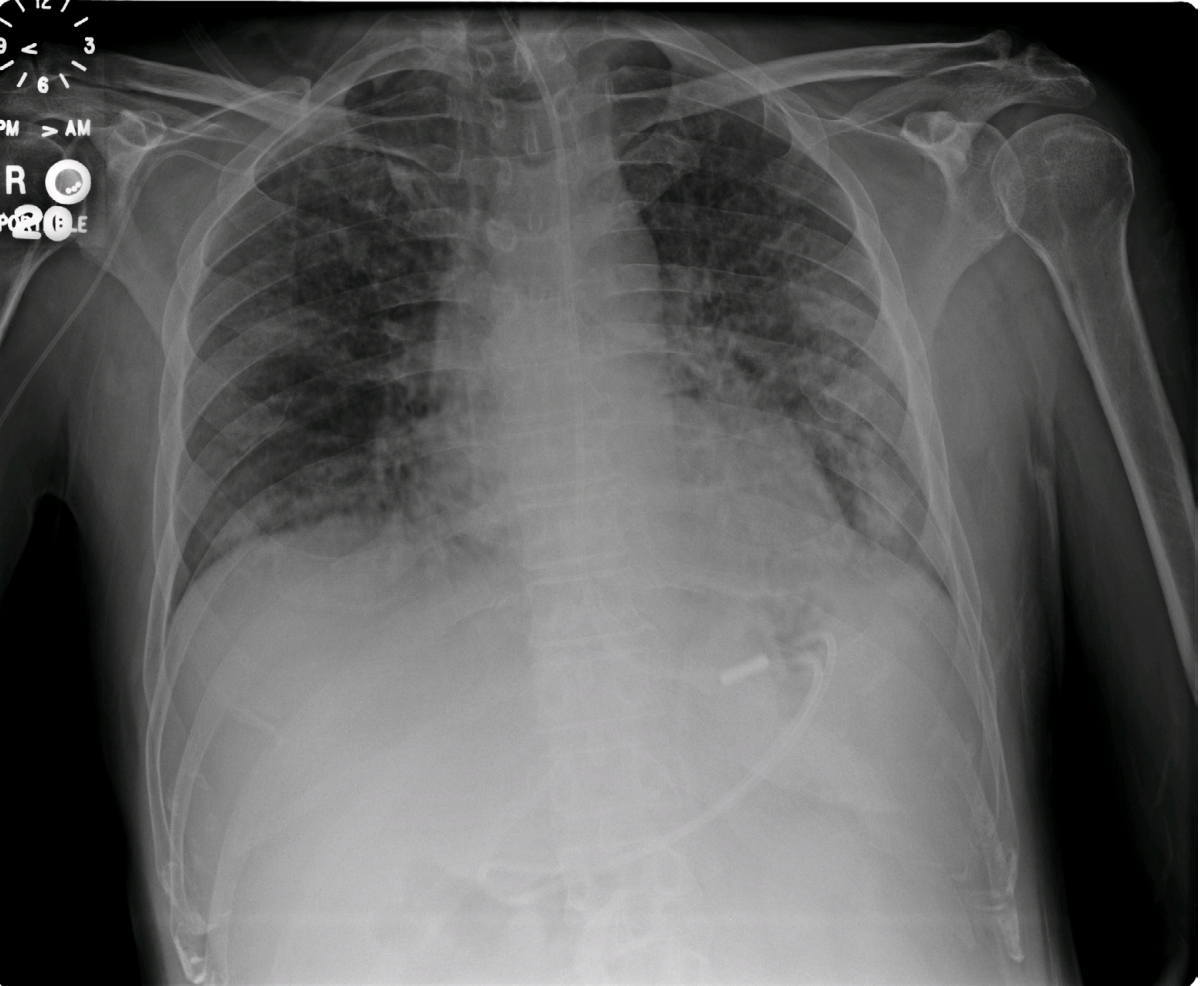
Antero-posterior view of the chest x-ray, showing bilateral diffuse alveolar opacities.
